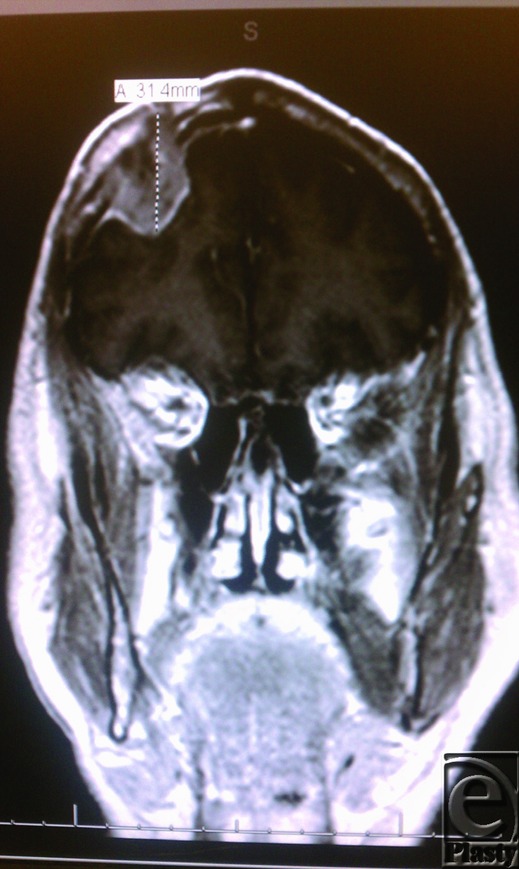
Left image: Magnetic resonance imaging coronal image of the head demonstrating enhancing mass of the right frontal bone with epidural extension into the extra-axial space overlying the right frontal lobe with mild associated mass effect.
